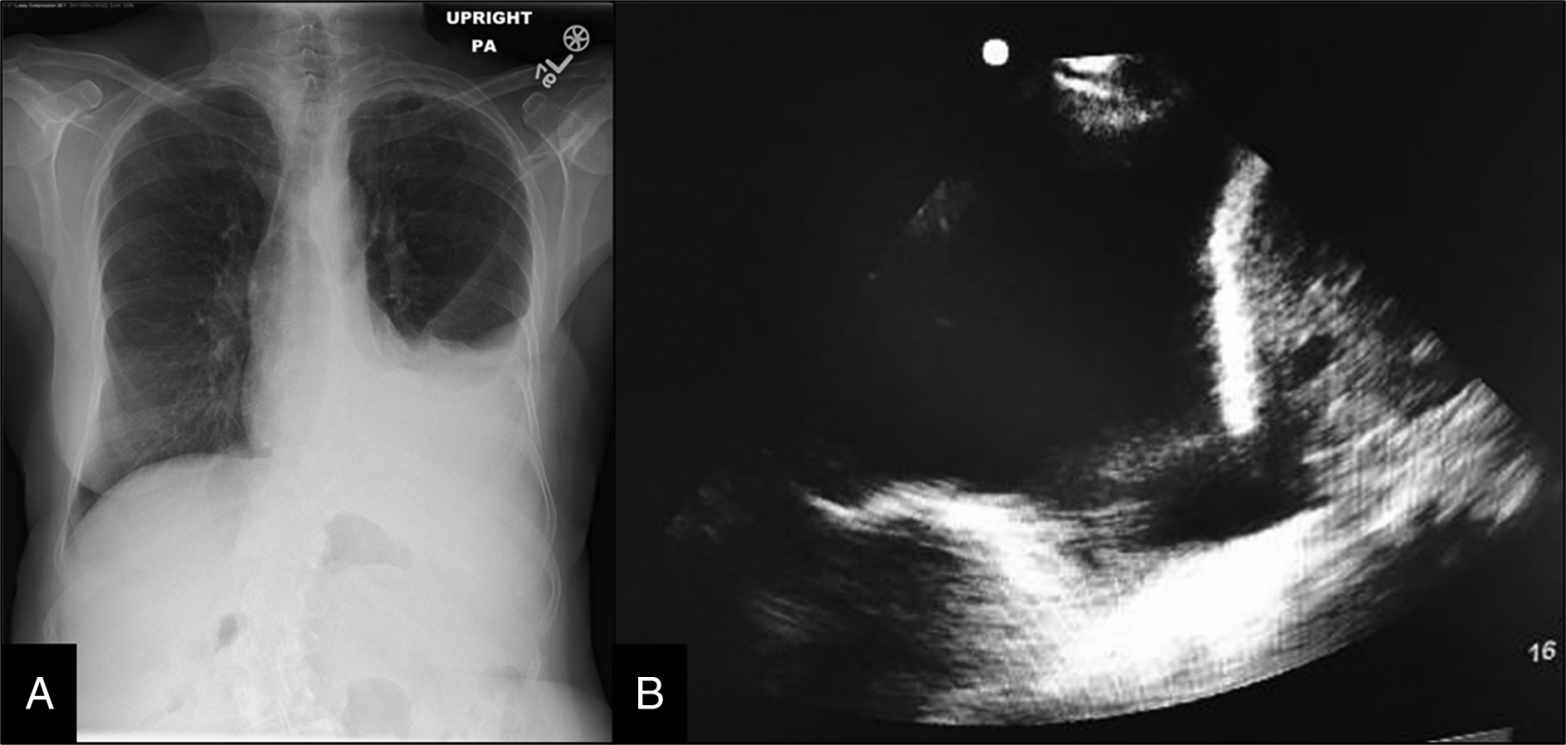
(a) Chest X‐ray demonstrating left‐sided pleural effusion. (b) Ultrasound of the left thorax showing pleural effusion.
